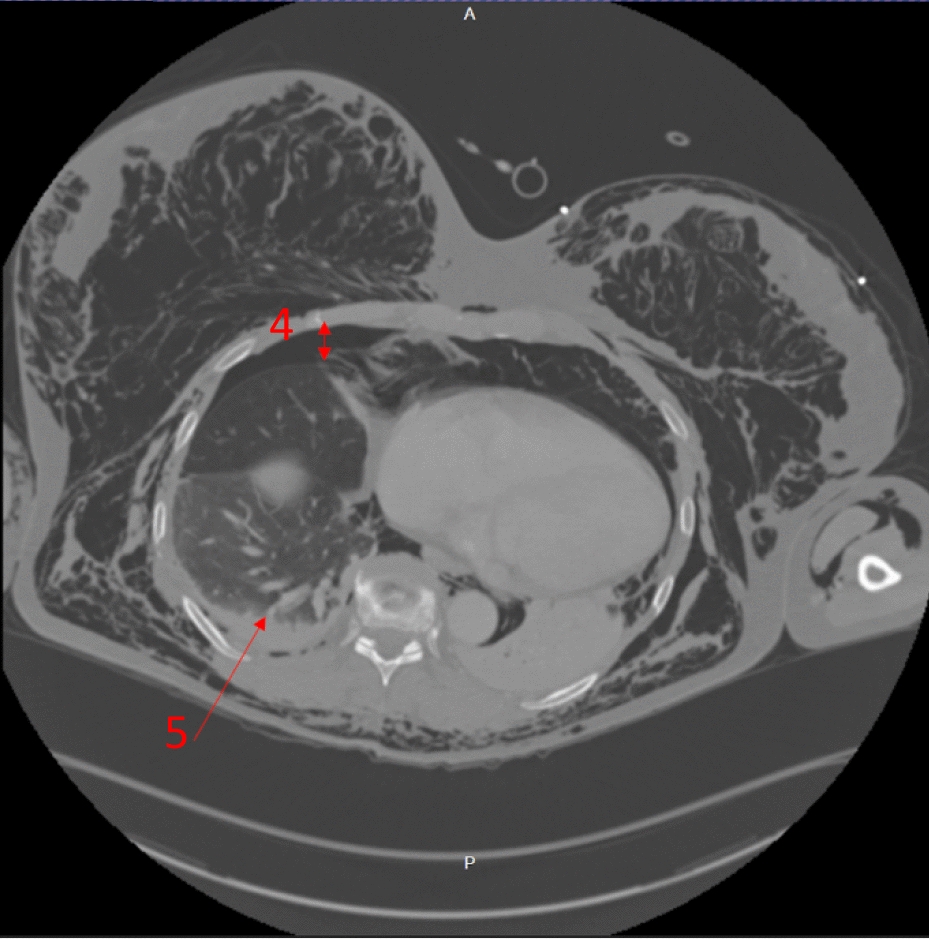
Axial computed tomography showing the right-sided moderate-volume pneumothorax (arrow 4) and the right-sided lower-lobe pulmonary hemorrhage (arrow 5)
